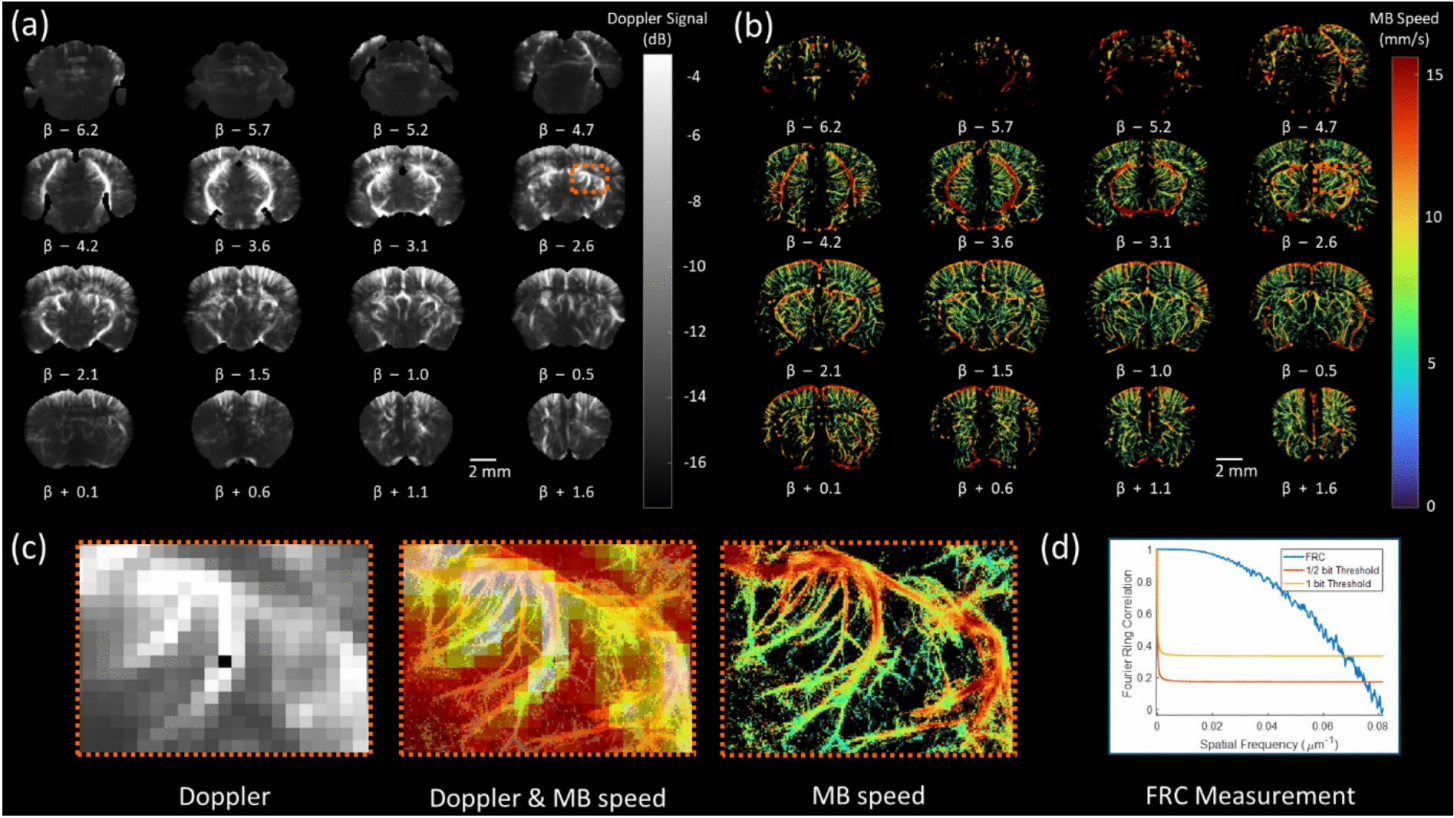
Comparison between Doppler image and ULM speed maps, covering a field of view of 11.9 × 7.87 × 8.1 mm3 across 16 imaging planes. β represents the z-position of each imaging plane with respect to bregma. (a) Time-averaged Doppler images highlight major vascular structures at a resolution of 110 × 98 × 525 µm. The colorbar represents the measured Doppler signal in dB. (b) Microbubble speed maps provide a more detailed representation of microvascular features and blood flow speed at 5 × 5 × 525 µm resolution. The colorbar represents the measure MB speed in mm/s. (c) The combined Doppler and ULM images acquired on the same imaging plane reveal additional vascular structures captured by ULM. (d) Fourier ring correlation analysis on ULM image, demonstrates a resolution limit of 13.33 µm.
